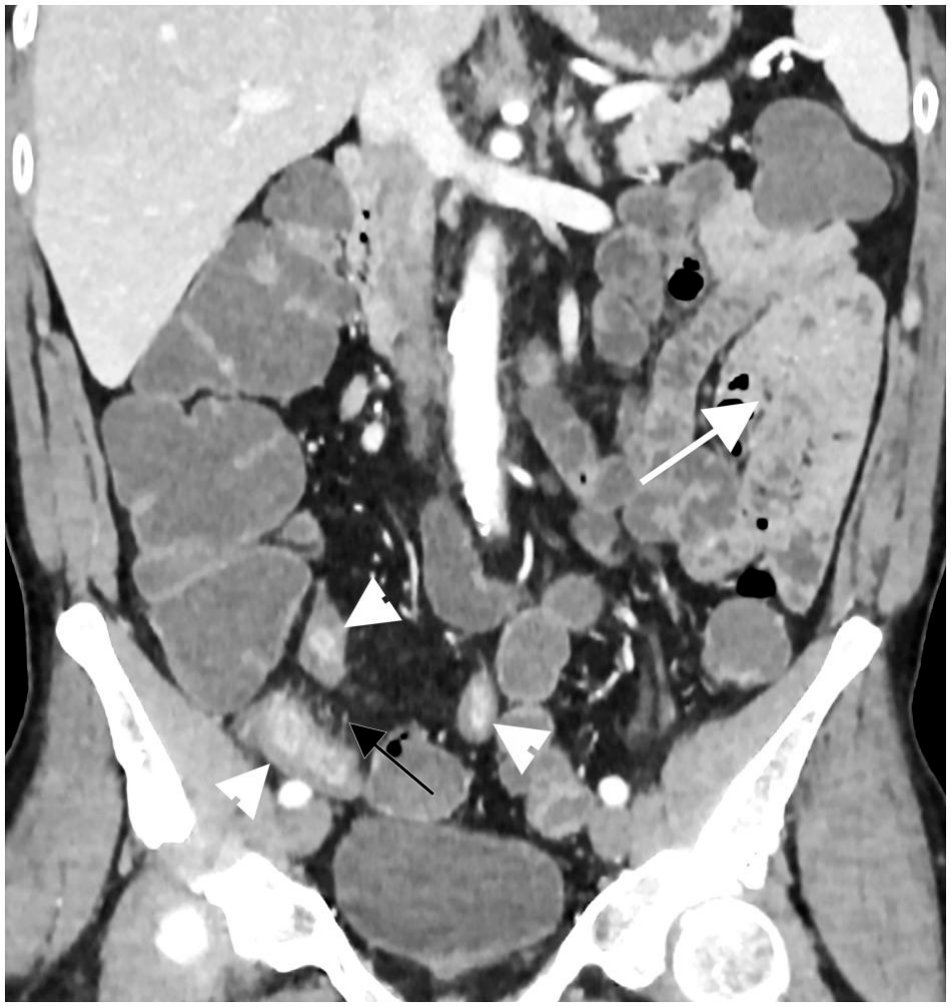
Example of not-good small bowel distension in CT-enterography in a patient with known Crohn’s disease: coronal CT, performed after iodinated contrast medium injection, shows how jejunal loops (white arrow) are not well distended from the oral contrast agents. Some pathological ileal loops are also seen in the right lower abdominal quadrant (white arrowheads) with wall thickening and stratified contrast enhancement; hypervascularity of the involved mesentery is also evident (black arrow).
